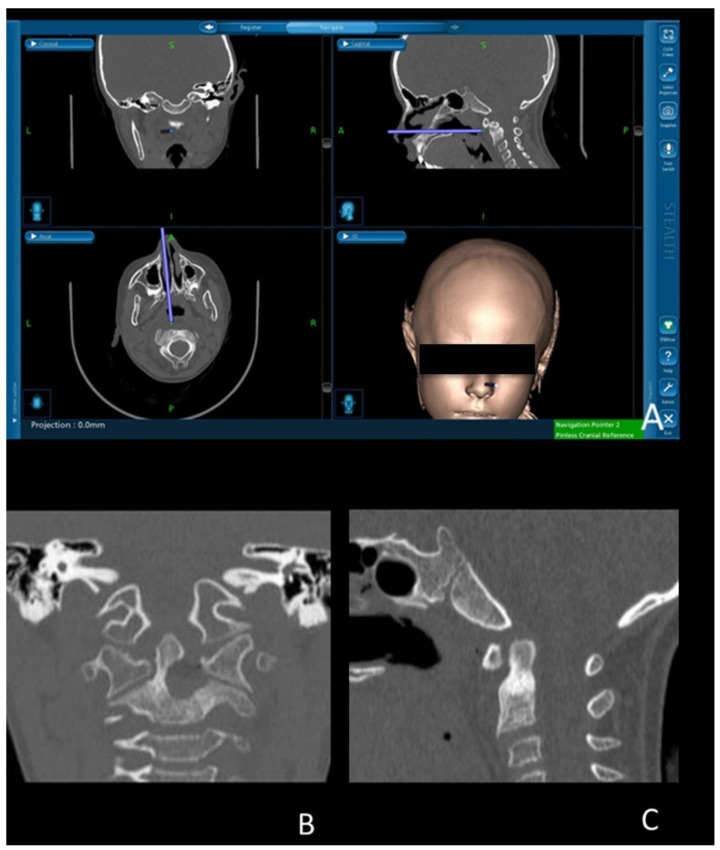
(A) Intraoperative neuronavigation during lesion removal; (B,C) Post-operative cervical CT scan showing the complete removal of C2 osteoid osteoma through transnasal endoscopic approach, with satisfactory sparing of the healthy odontoid process. Purple line: intraoperative surgical trajectory.
